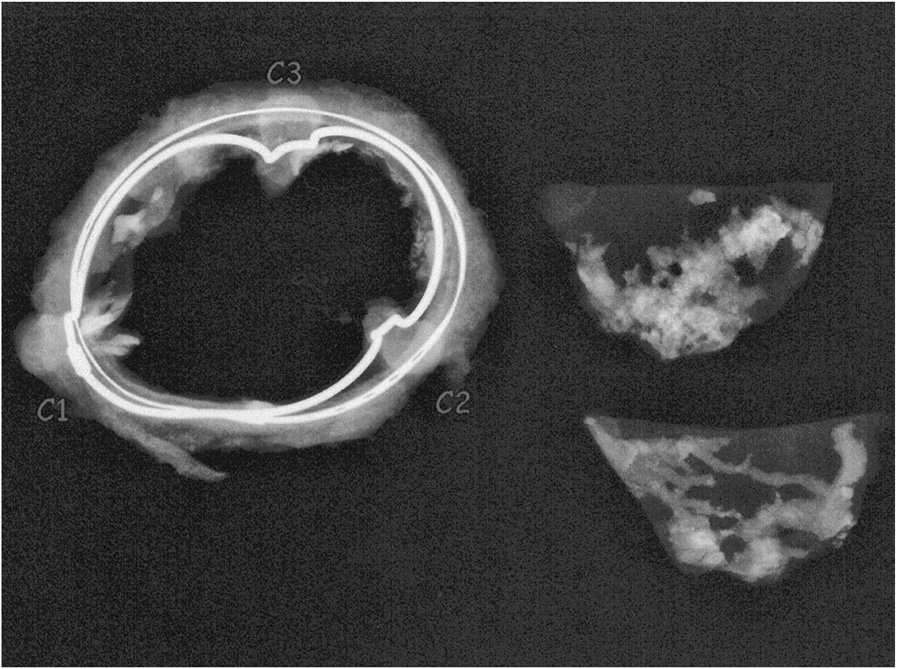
Acinar and linear calcifications are found in the cusps by X-ray examination.
